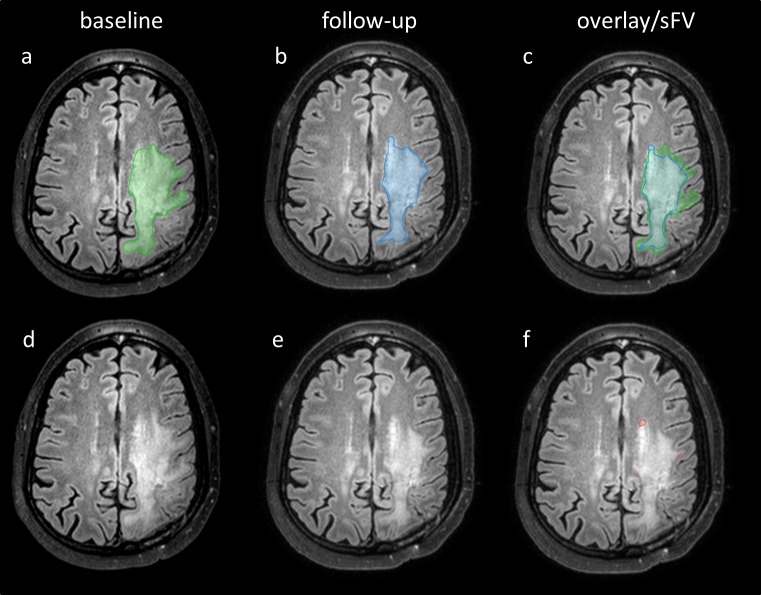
Examples of FLAIR segmentations and regional, segmentation-based subtractions. Axial FLAIR slices of the baseline MRI (a, d) and the follow-up MRI (b, c, e, f) of a patient with GB are shown. Segmentation of baseline FV (a, green), follow-up FV (b, blue) and an overlay image of both segmentations (c) is presented. Baseline FLAIR image (d) and follow-up FLAIR image (e) are displayed without segmentations. The regional, segmentation-based subtraction of FV (sFV) is also shown (f, red).
